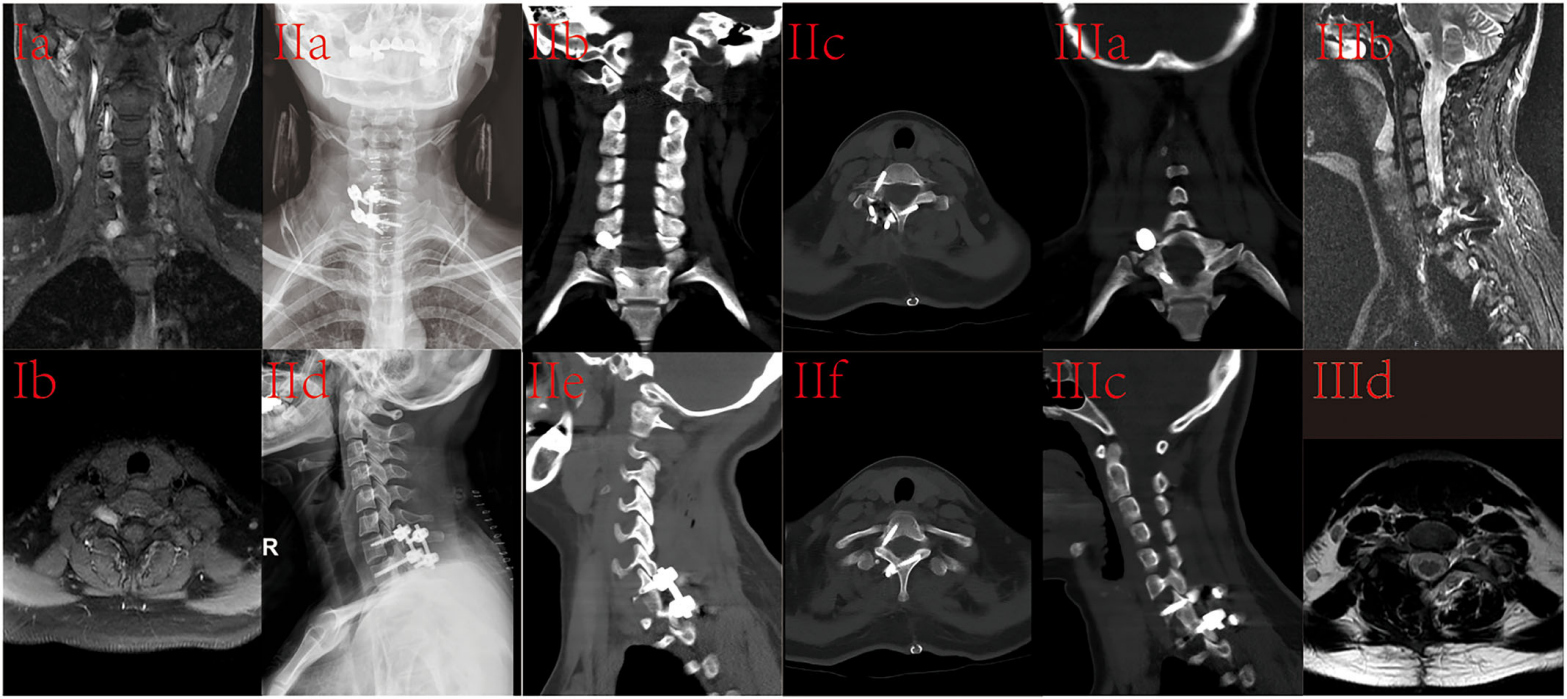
A 27‐year‐old female was diagnosed with a C7/T1 spinal tumor. The preoperative magnetic resonance imaging (MRI) coronal and cross‐sectional views (IA‐B) showed a tumor at the right intervertebral foramen of C7/T1. Anteroposterior and lateral radiographs (IIA, IID) and computed tomography (CT) coronal and sagittal views (IIB, IIE) 3 days after surgery showed the implant in place, and no fusion of the C7‐T1 lateral mass graft bone. Good positioning of the pedicle screws + pedicle screws in the C7 and T1 segments can be seen in transverse CT views (IIC, IIF). At 3 months after the operation, CT coronal and sagittal views (IIIA, IIIC) showed fusion of C7‐T1 lateral mass graft bone, and MRI coronal and cross‐sectional views (IIIB, IIID) showed complete resection of the tumor and no evidence of recurrence.
